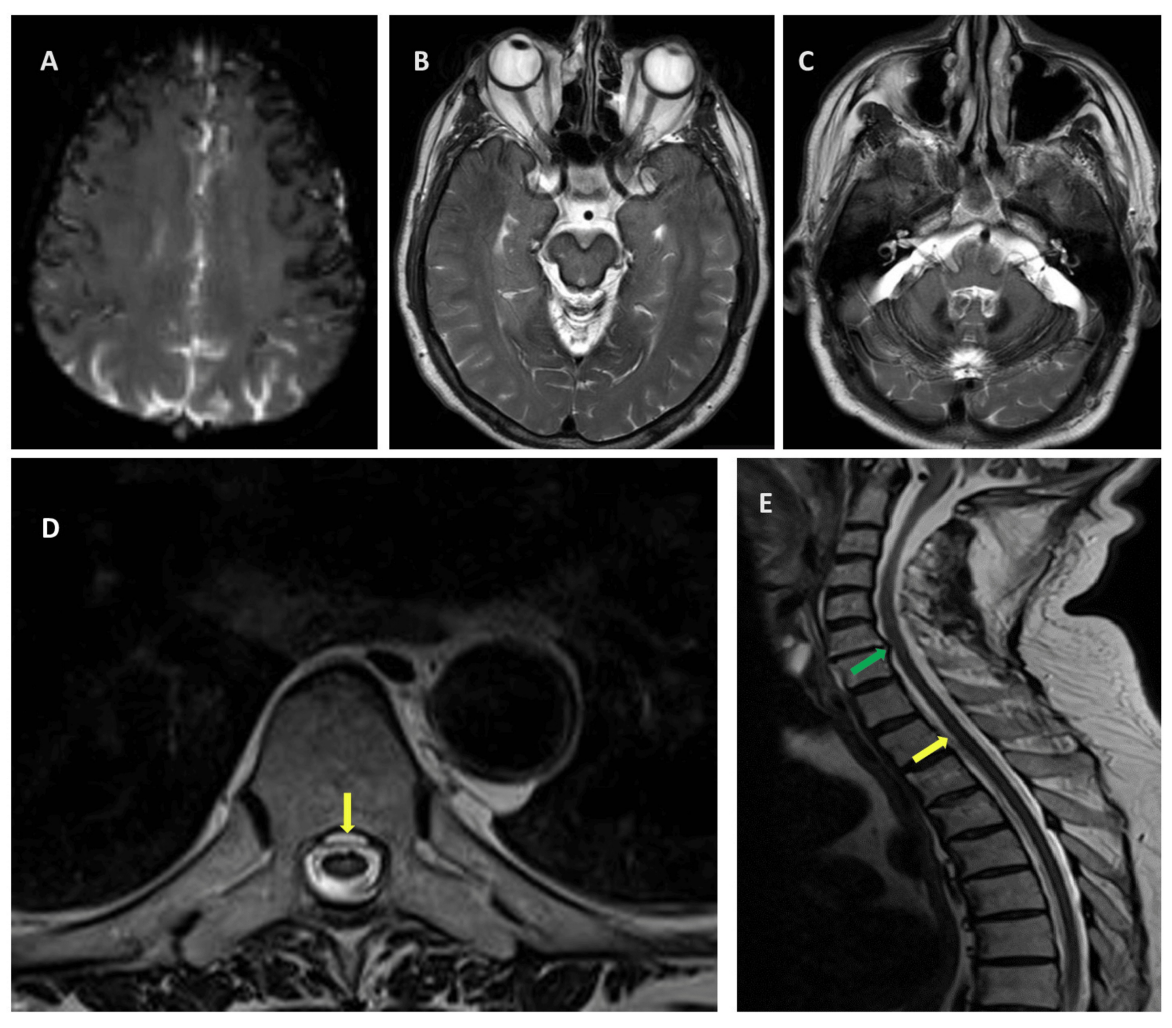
The axial and sagittal brain and spinal MRI from patient Axial gradient echo (A) and T2-weighted (B-C) brain MRI showing signal hypointensity due to hemosiderin deposition along the cerebral convexities (A), around the midbrain (B), and along the cerebellar folia (C). The T2-weighted axial (D) and sagittal (E) images of the cervical and thoracic spine showed ventral epidural fluid collection extending from C6 to T10 (yellow arrows) and disc-osteophyte complex at the C6-7 disc level (green arrow).
